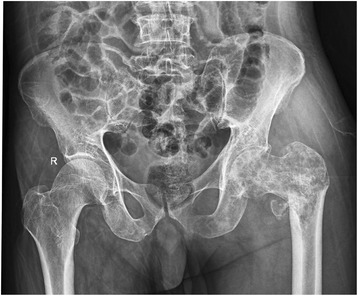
Anteroposterior view of X-ray pelvis suggesting multiple metastasis and pathological fracture of left hip
